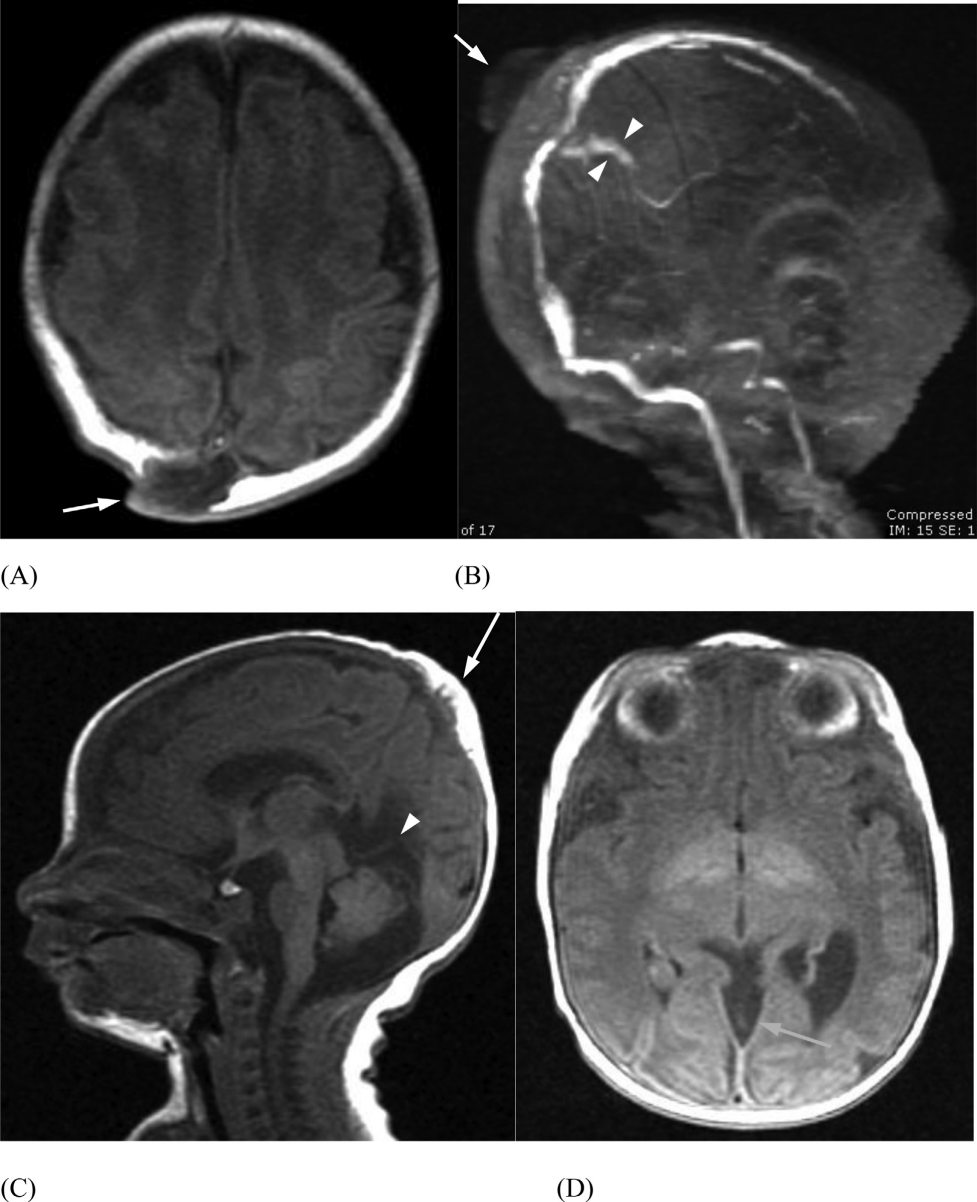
(Case 2) (A) Axial T1 demonstrates the atretic parietal cephalocele (APC) immediately posterior to the superior sagittal sinus (white arrow). (B) MRV demonstrates persistent vertical falcine sinus (arrowheads) extending superiorly to the APC (white arrow). (C) Sagittal T1 shows prominence of the superior cerebellar cistern, peaking of the tentorium (arrowhead) and the atretic parietal cephalocele (white arrow). Axial MRI (D) demonstrates an enlarged temporal horn of the left lateral ventricle and a prominent peri-pineal recess (blue arrow).
